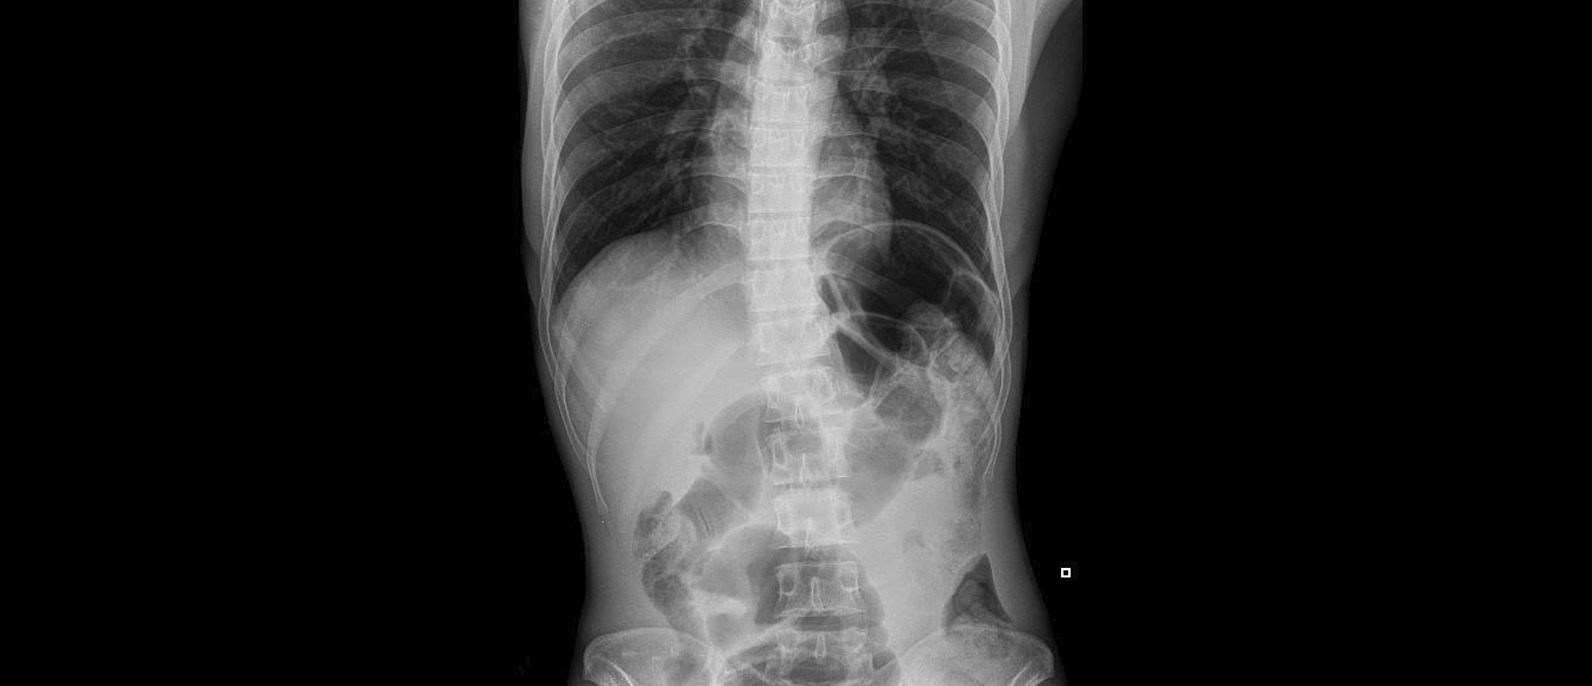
Plain erect abdominal radiograph showing distension of colon in the right lower quadrant-pelvis region
